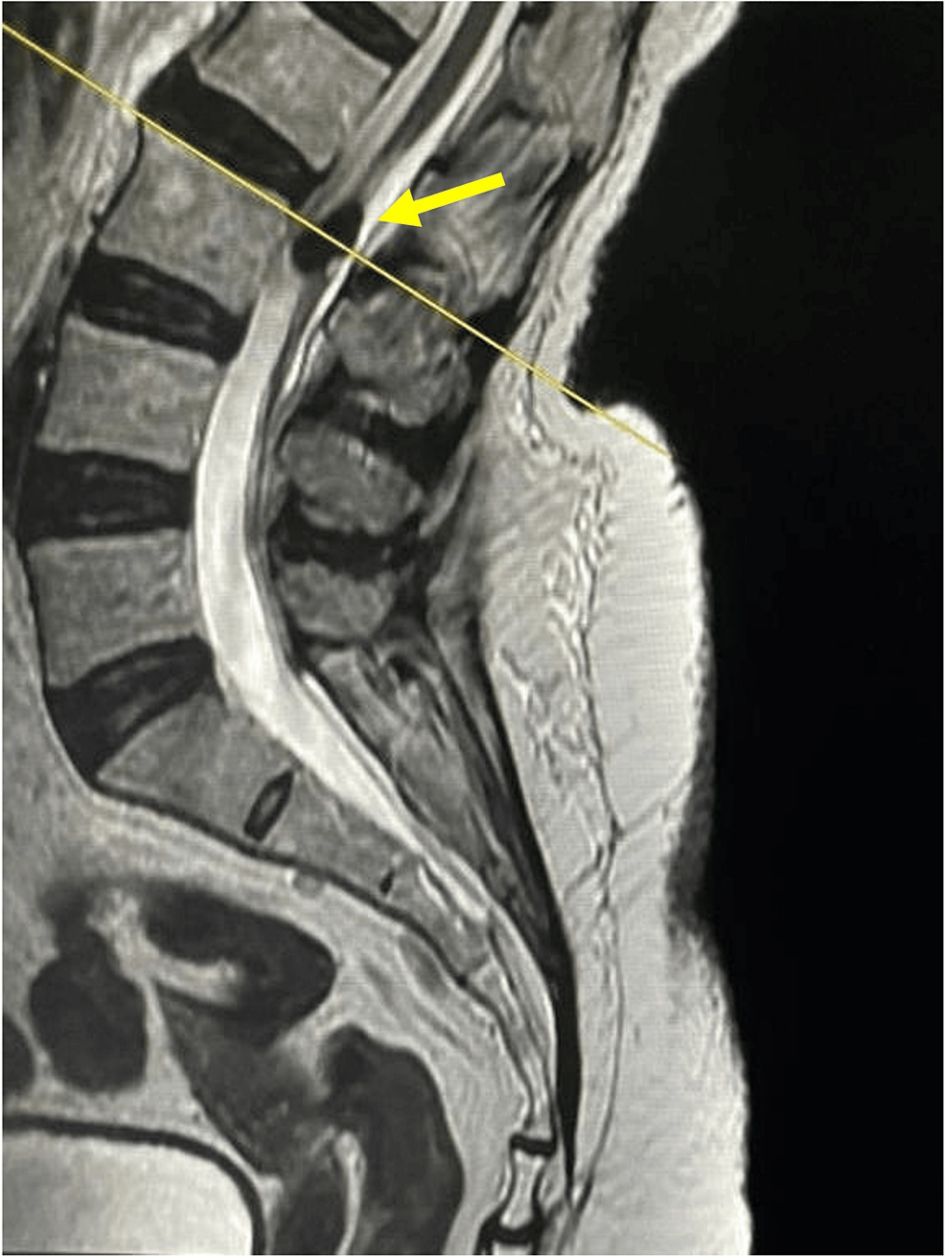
Preoperative imaging studies A sagittal T2 sequence MRI shows a tumor in L2-L3 intervertebral space (yellow arrow).
